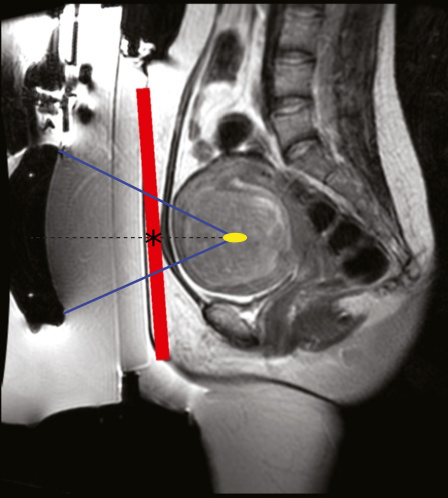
Sagittal image of the MRgFUS setup showing the uterine fibroid, focused ultrasound transducer within the oil bath, and coupling gel pad. Placement of the T2 mapping slice (*red*), the transducer axis (*dotted line*), and intersection of the axis and the slice (*asterisk*)
